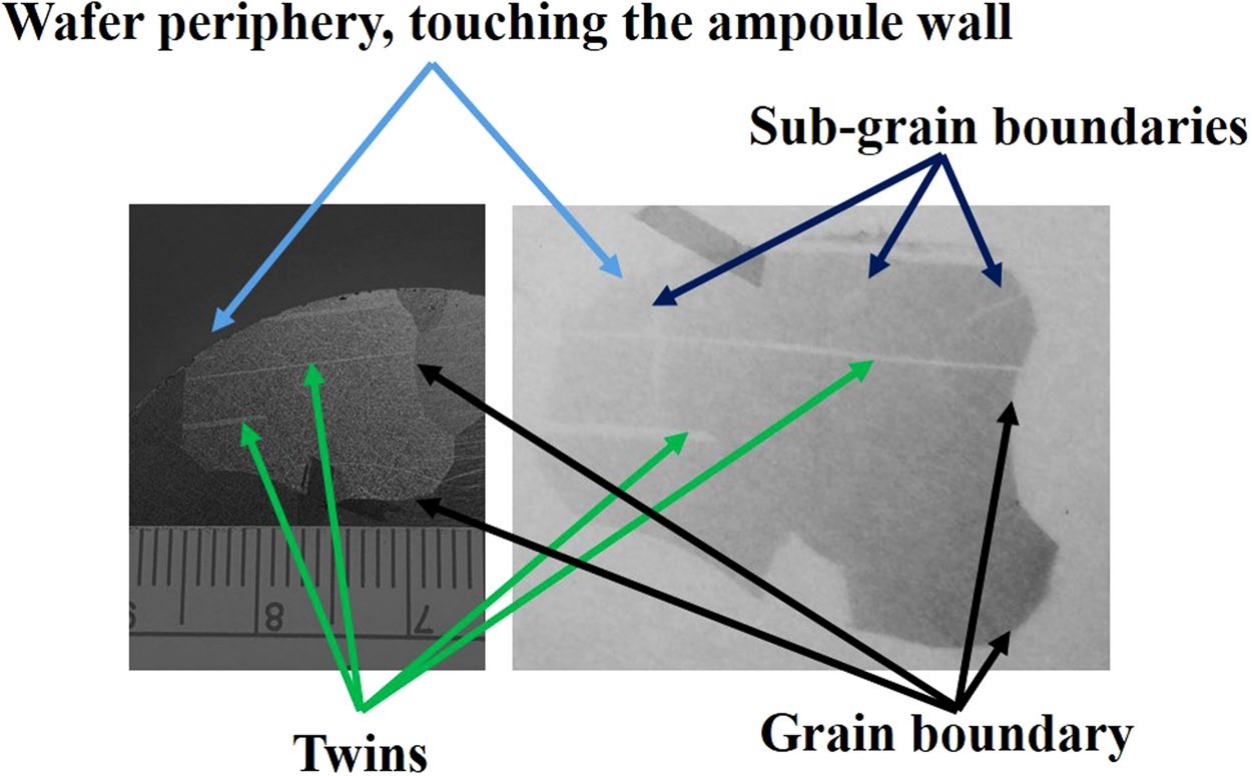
Optical photograph of the grain (left) and the corresponding X-ray topographic image of the grain.
